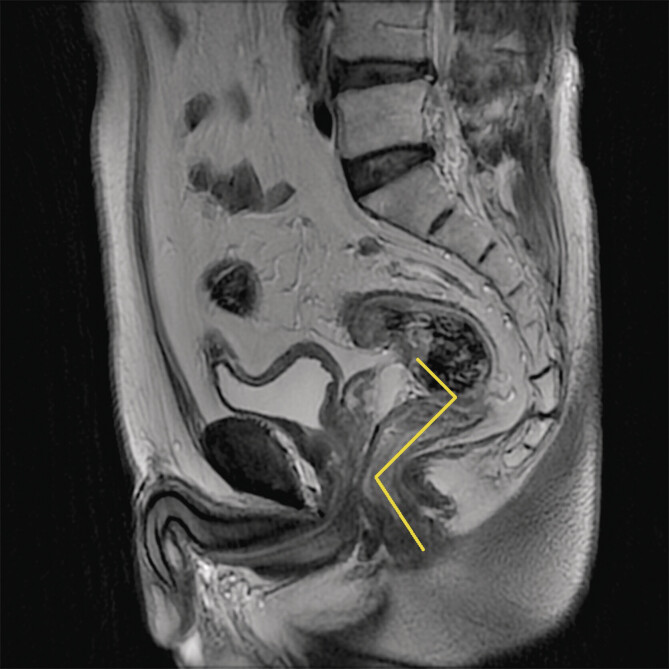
On sagittal T2-weighted MRI images, the DTAV is measured by
